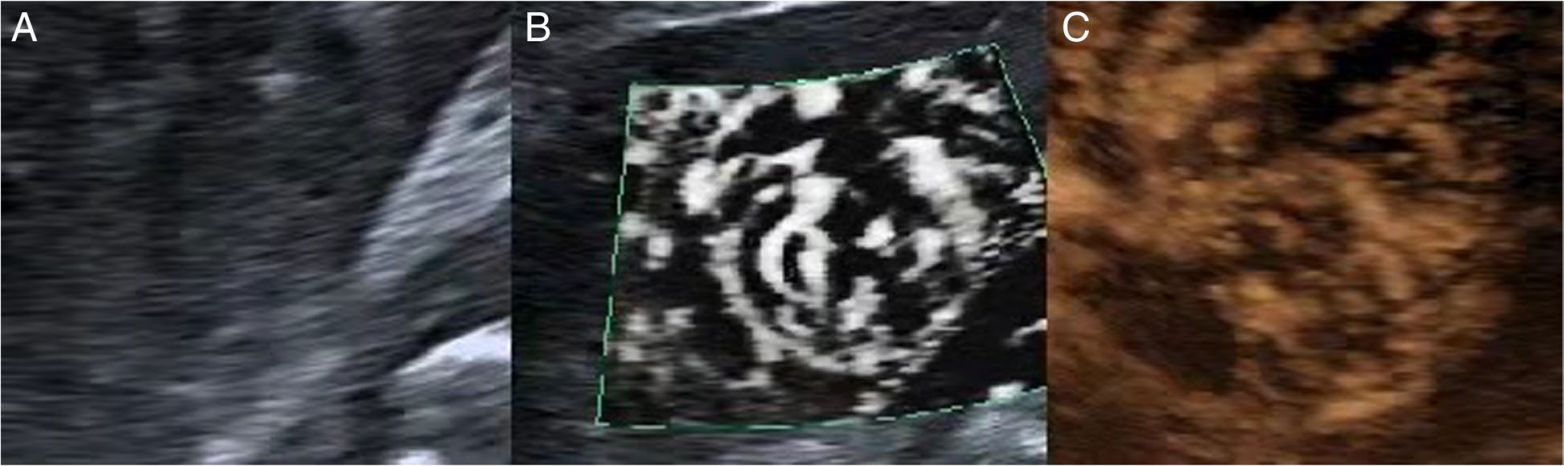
A solid tumor of the right kidney A ultrasound imaging, hypoechoic mass with unclear boundary, regular shape, and inhomogeneous internal echo B SMI show multiple large vessels and their dot and linear blood flow branches around and inside the tumor, blood flow grade 3 C MFI ultrasonography showed more clearly vascular branches than SMI, and it could show the branch shape. blood flow grade 3. The pathological result was clear cell renal cell carcinoma
